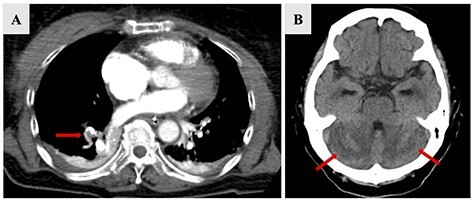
Chest CT with contrast revealed filling defects of the right peripheral pulmonary artery (A, arrow). The head CT on Day 5 revealed hypodensities in the bilateral cerebellar hemispheres (B, arrow).
